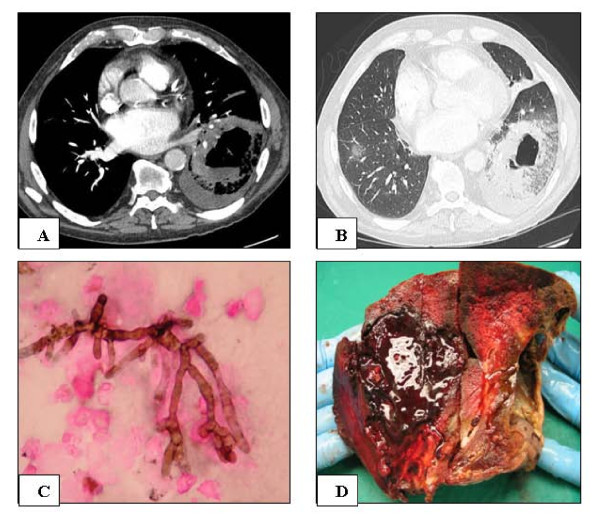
A and B: CT scan showing a large excavated pulmonary mass of the pulmonary left inferior lobe, outlined by a glassy feature and associated with pleural effusion (A. Non injected CT scan; B Injected CT scan). C. Mycotic filaments showing the morphological features of aspergillosis isolated from the BAL (Gomori-Grocott silver method × 1000). D. Gross macroscopy of the resected pulmonary lobe showing a hemorrhagic infarct.
